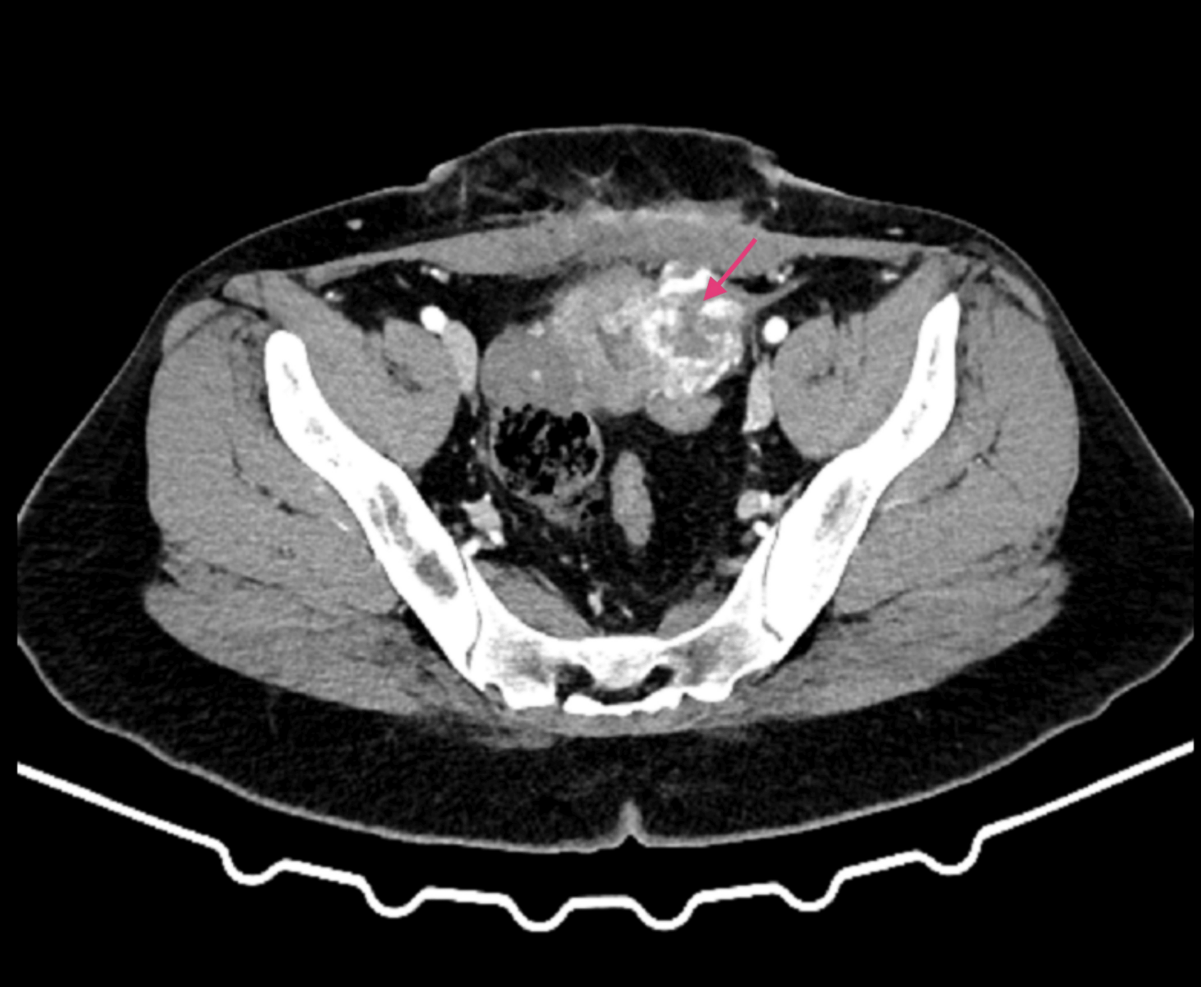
Computed tomography (CT) demonstrating a nodular mass in the uterine body (pink arrow)
